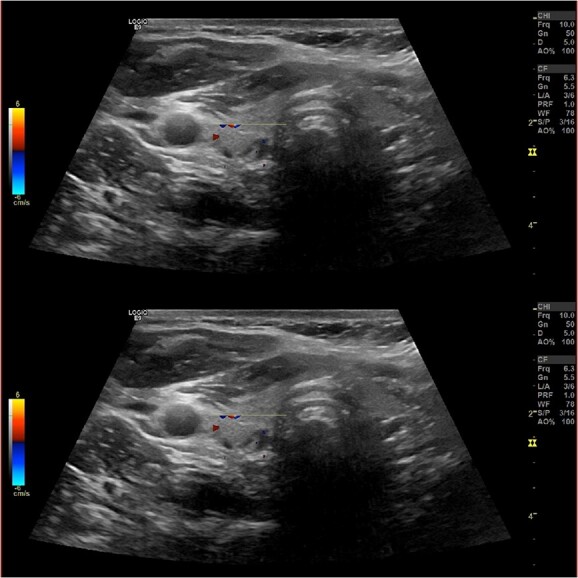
Ultrasound neck showing normal thyroid gland and no abnormal parathyroid.
